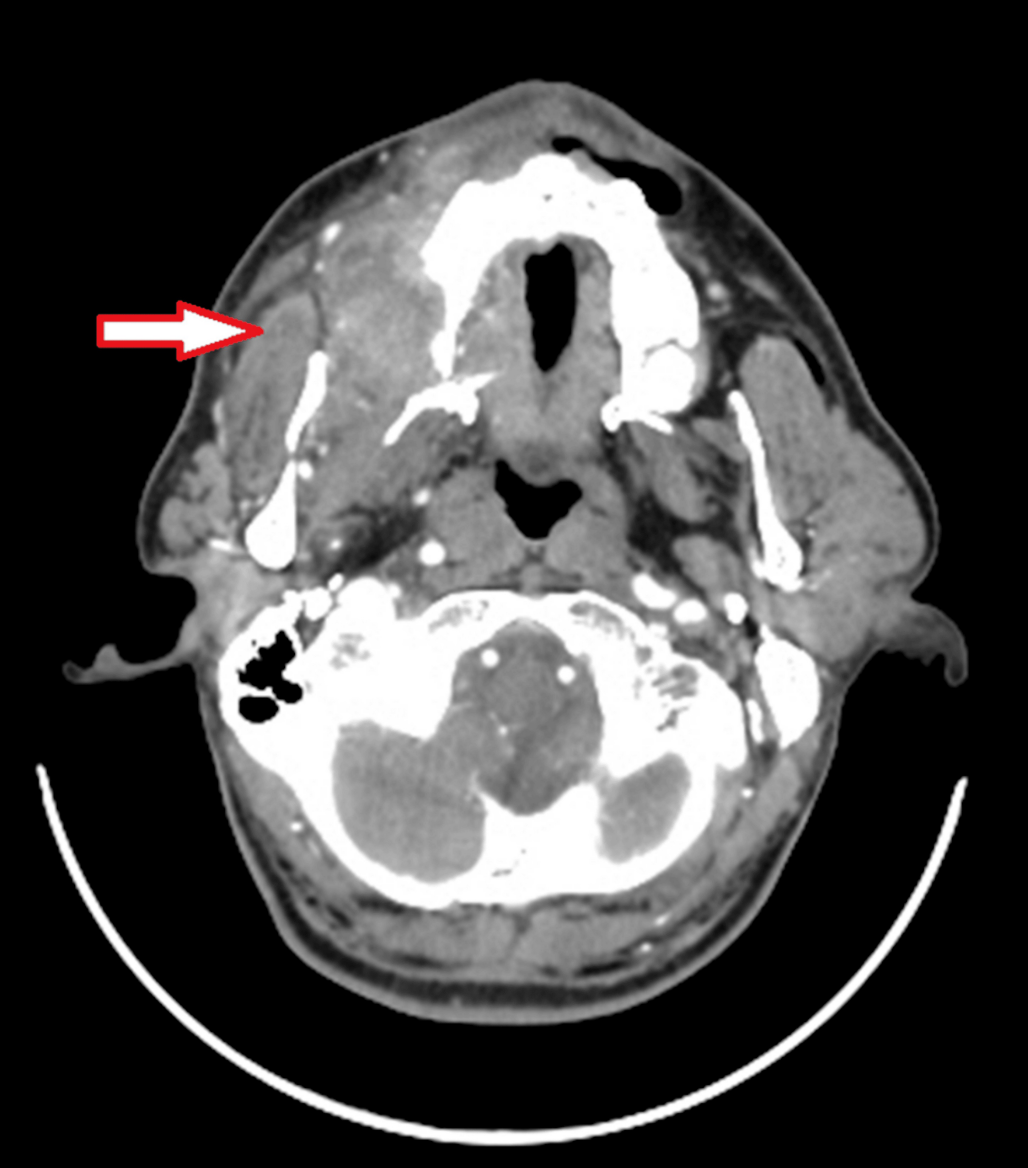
Contrast-enhanced computed tomography image of the lesion
